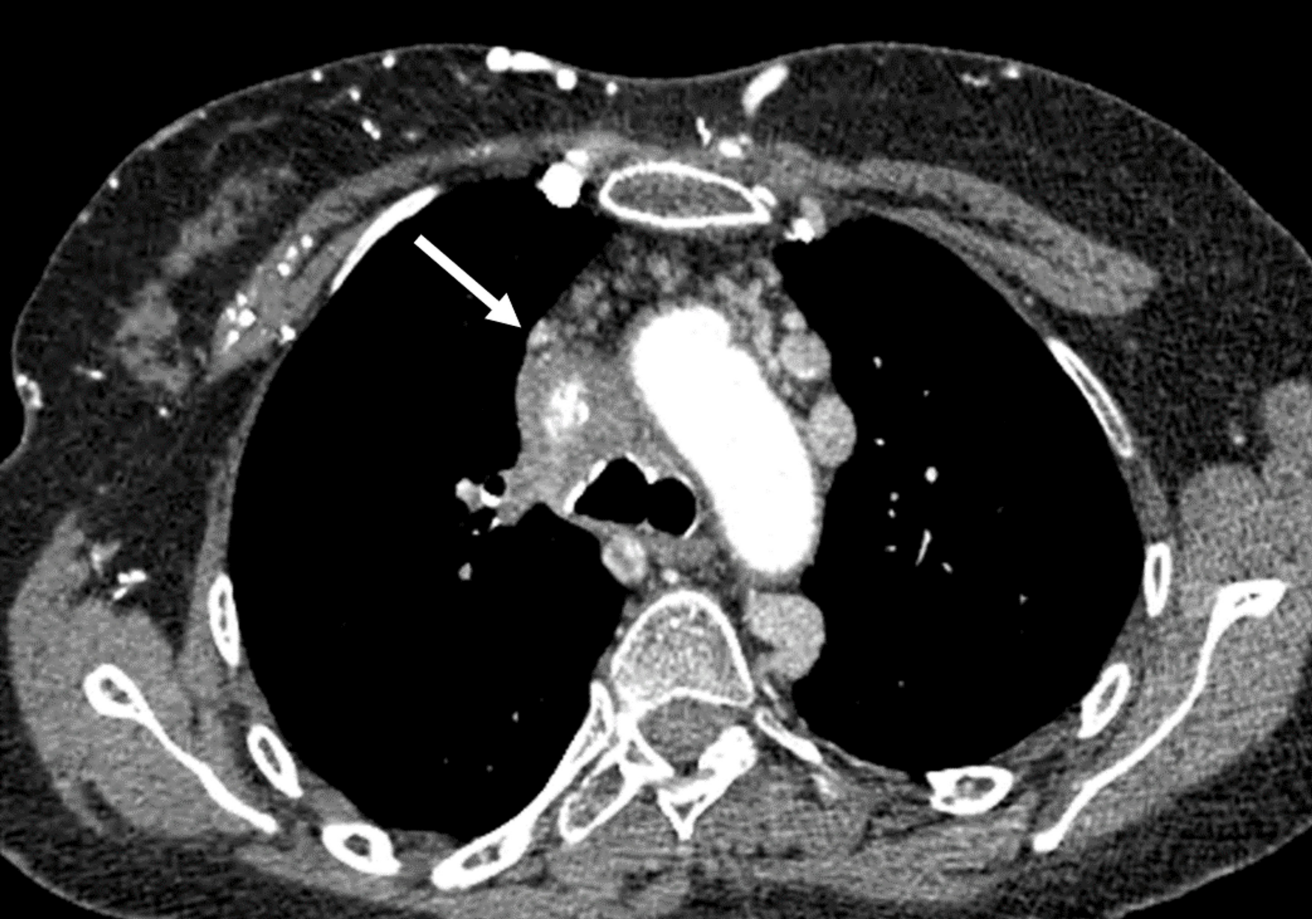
Chest CT scan, mediastinal window, axial section after iodinated contrast injection with acquisition at 35 seconds: Circumferential parietal thickening of the SVC + CVC thoracic wall SVC: superior vena cava; CVC: collateral venous circulation
